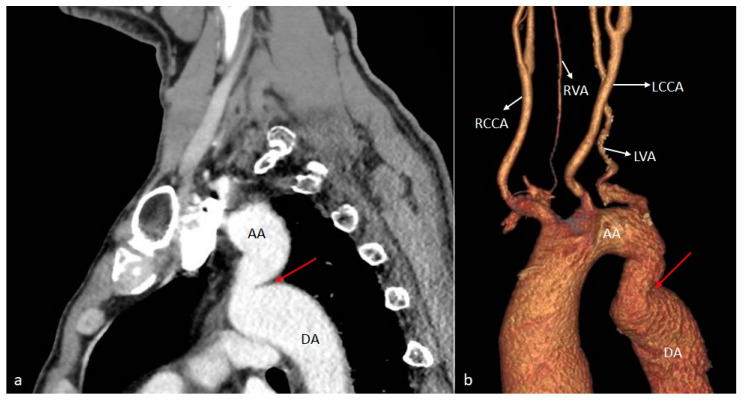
MPR sagittal (a) and VR (b) CT images show a periductal narrowing and kinking of the aorta (red arrows) without the subsequent dilation of the supra-aortic trunks, intercostal and internal mammary arteries, findings suggestive of the pseudocoarctation of the aorta. AA—aortic arch. DA—descending aorta. RCCA—right common carotid artery. LCCA—left common carotid artery. LSA—left subclavian artery. RVA—right vertebral artery. LVA—left vertebral artery.
